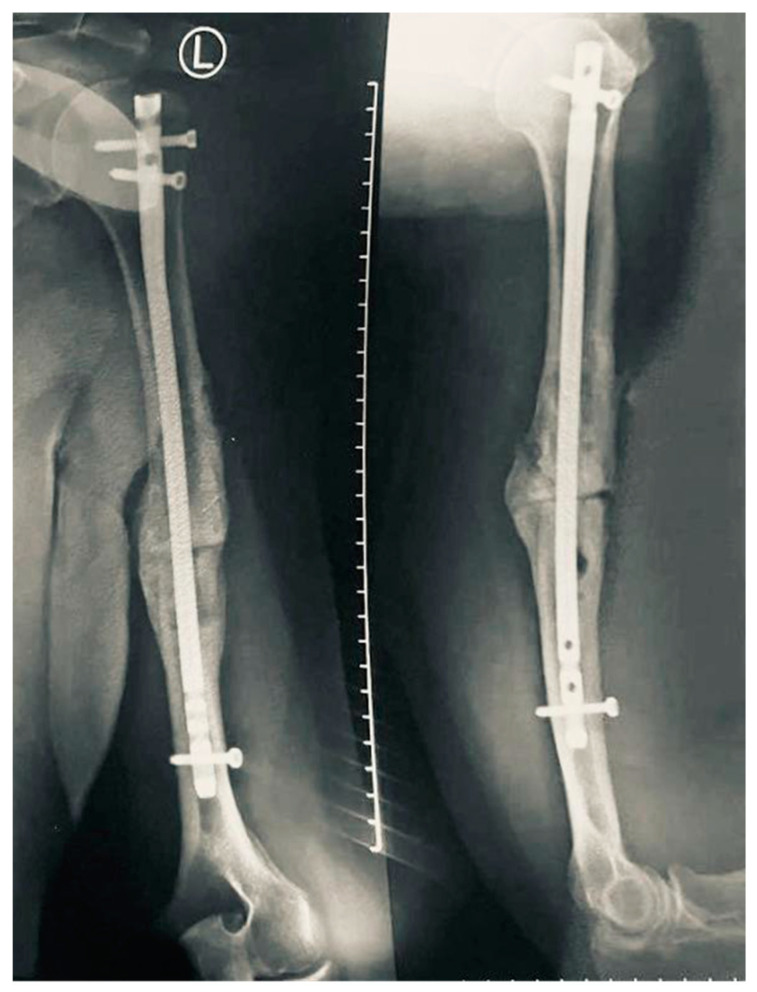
X-ray at six-month follow-up. Left: antero-posterior, and right: latero-lateral view, showing fracture consolidation in process.
